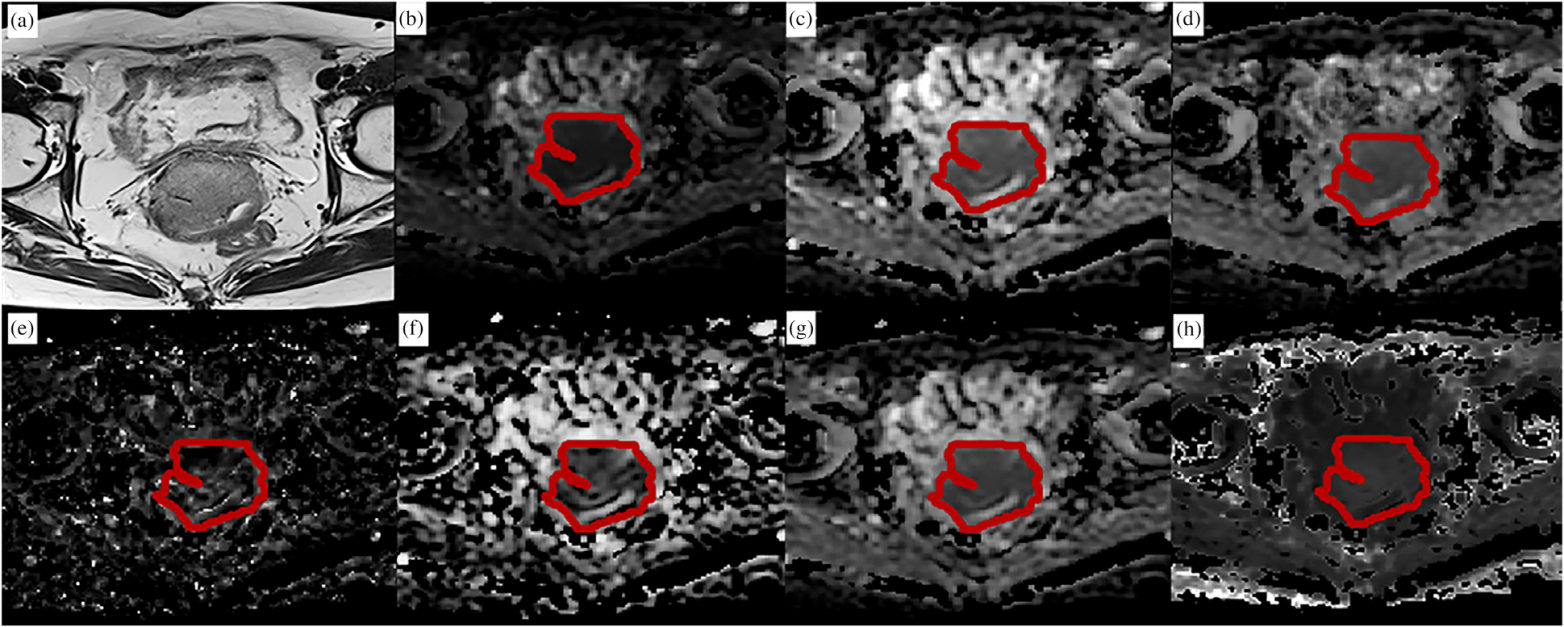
A 57‐year‐old patient with International Federation of Gynecology and Obstetrics (FIGO) stage IIB cervical cancer magnetic resonance imaging (MRI), marked with a region of interest (ROI). (a) T2WI, (b) tumor was manually drawn at the diffusion‐weighted imaging (DWI) image at *b* = 800 s/mm^2^, (c∼h) are copied contour to corresponding ADC map, D map, D* map, f map, MD map, and MK map, respectively. The average values (ADC∼MK) of the region of interest (ROI) are 900.10 × 10^−3^ mm^2^/s, 900.49 × 10^−3^ mm^2^/s, 1225.57 × 10^−3^ mm^2^/s, 93.5%, 1216.58 × 10^−3^ mm^2^/s and 854.99, respectively.
